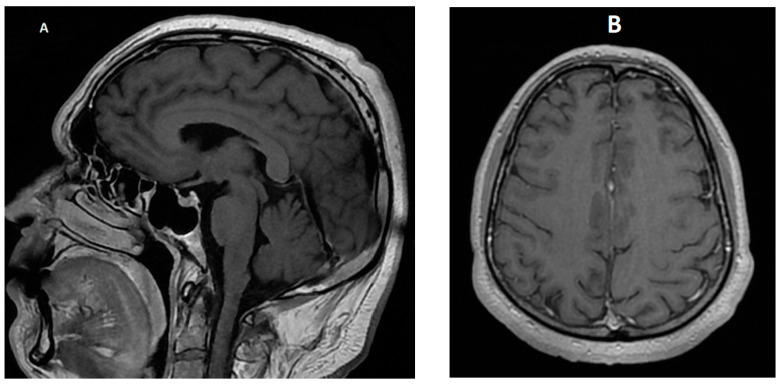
Normal MRI imaging of the brain on follow-up. (A)—sagittal contrast-enhanced T1-weighted image; (B)—axial contrast-enhanced T1-weighted image.
